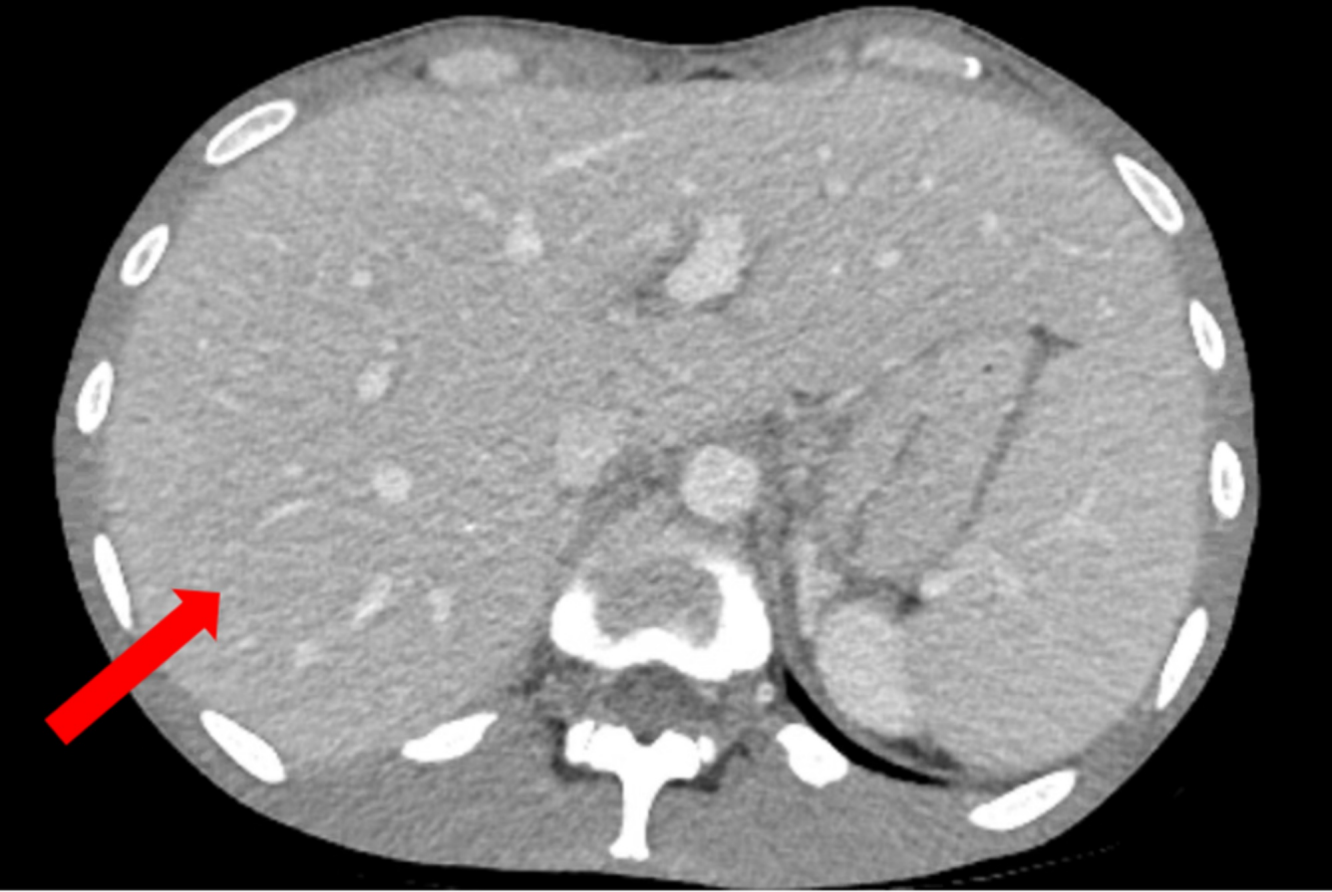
CT of the abdomen with IV contrast The image shows hepatomegaly (red arrow) CT: computed tomography; IV: intravenous
